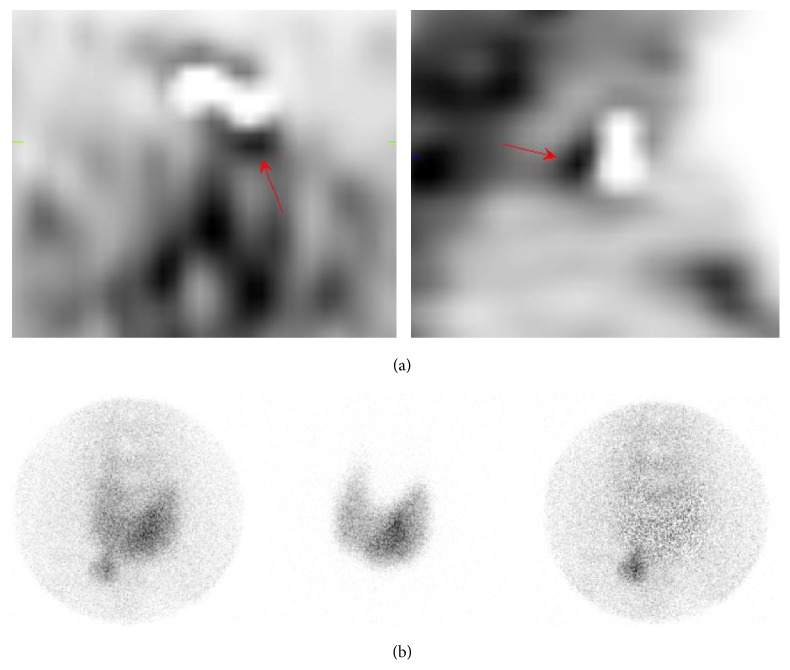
An example of a false-positive finding (case number eight in Table 3) on a ^99m^Tc-sestamibi/^123^I SPECT/CT due to an edge artifact (arrows). Oversubtraction is intentional to demonstrate the effect. An additional pinhole image showed no residual activity and was correctly classified as true negative. (a) Subtraction SPECT/CT images (*left*: transaxial;* right*: sagittal). (b) Anterior pinhole images (*left*: ^99m^Tc-sestamibi image;* middle*: ^123^I image;* right*: subtraction image).
